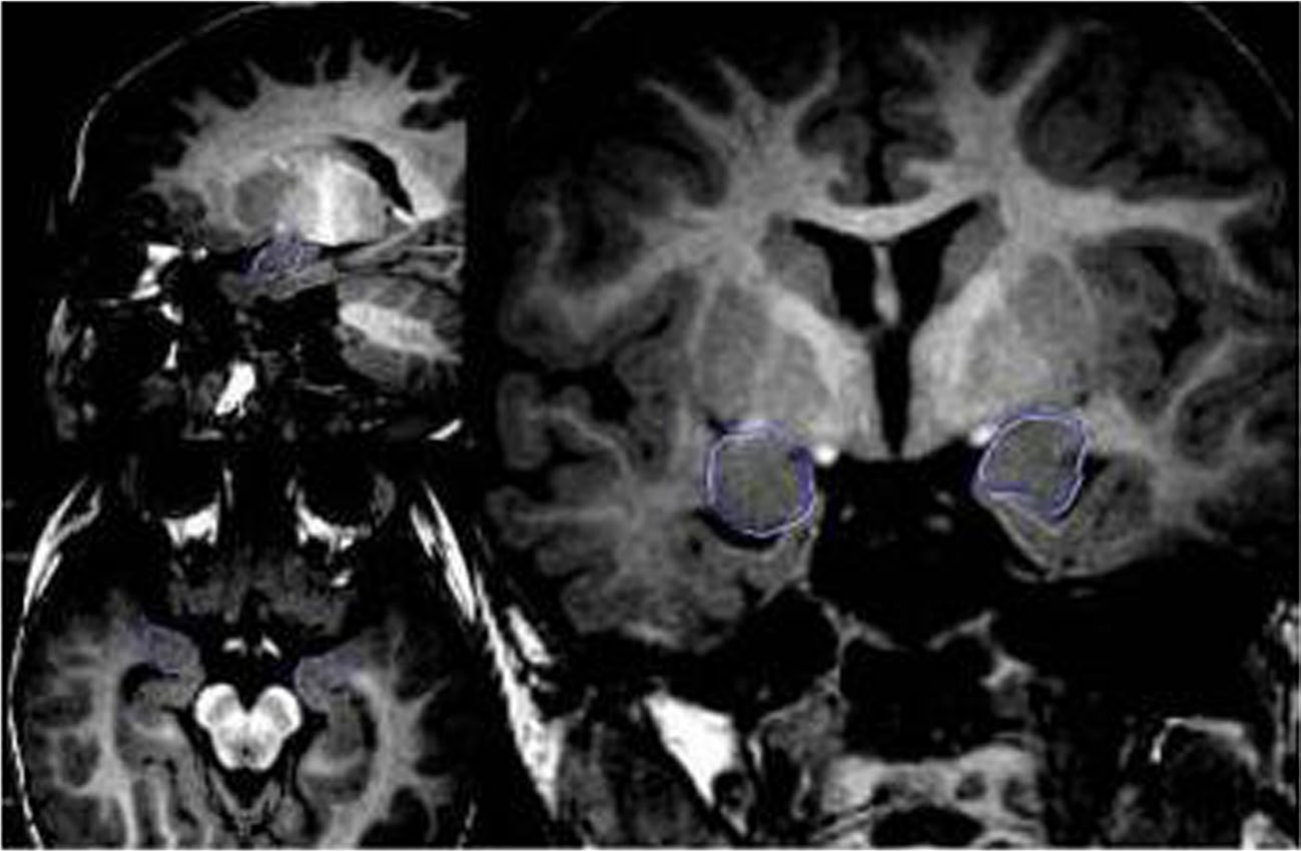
Sagittal, axial, and coronal view of T1WI on MRI sections. A three-dimensional reconstruction of images showed bilateral amygdala with the signal of gray matter and the reference lines for measuring the volume (blue circles)
